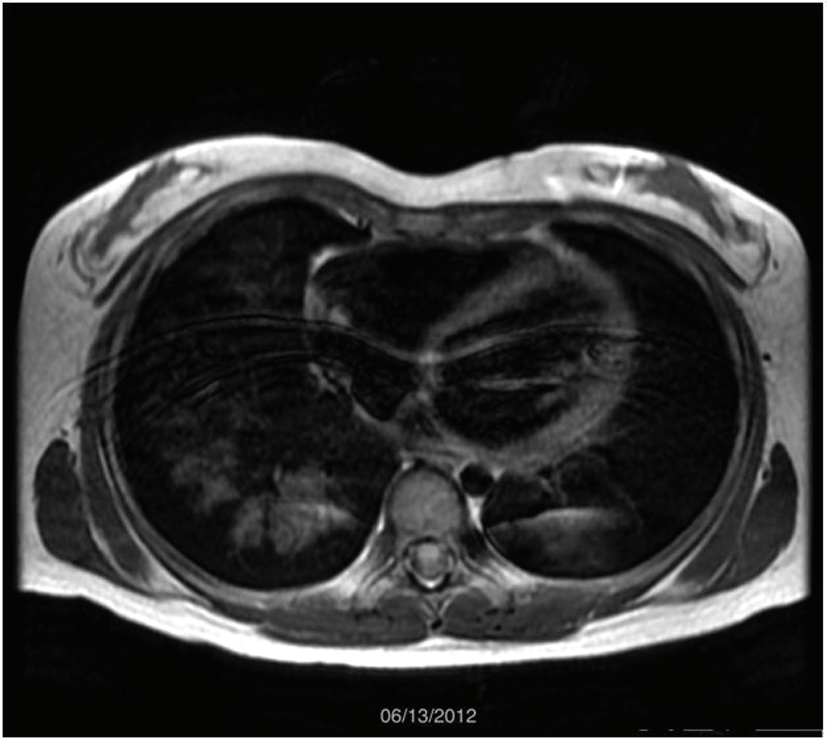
MRI scan obtained on June 2012 demonstrated diffuse airspace opacities in the right lower lobe and left lower lobe. Clinically, the patient was experiencing hemoptysis and found to be in an exacerbation of her condition.
